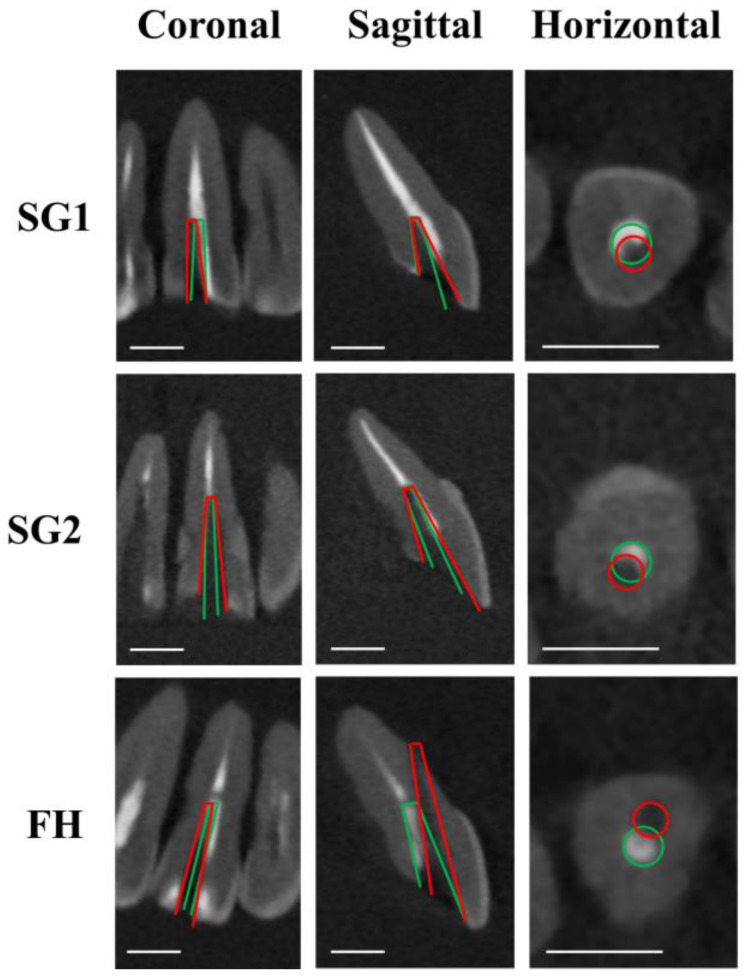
Representative CBCT images after fiber post removal on central incisors. Green lines show planned pathways, and red lines show actual drilling pathways. Scale bar = 5 mm.
